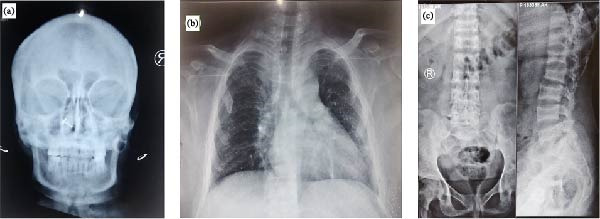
(a) Skull radiograph demonstrating calvarial thickening with a granular ‘salt‐and‐pepper’ appearance and subcortical resorption around the mandibular tooth sockets with loss of lamina dura. (b) Chest radiograph demonstrating diffuse bony rarefaction with an accentuated trabecular pattern and subchondral resorption at the costochondral junctions. (c) X‐ray of the lumbosacral spine (AP and lateral views) showing generalised osteopenia with biconcave vertebral bodies consistent with codfish vertebrae and reduction in vertebral body heights.
